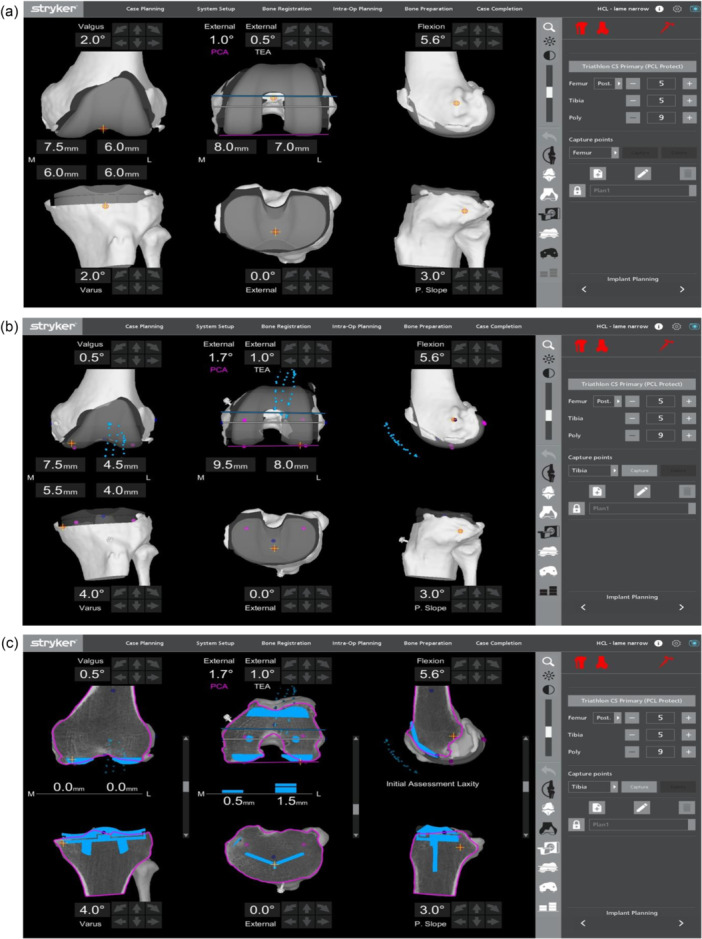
Ligament pillar. Intraoperative gap assessment and final implant positioning. (a) Preoperative CT‐based planning showing initial implant positioning and predicted bone resections. (b) Final implant positioning after adjustment according to extension and flexion gap assessment. (c) Final gap configuration demonstrating a tight and symmetrical extension space and controlled mediolateral asymmetry in flexion.
